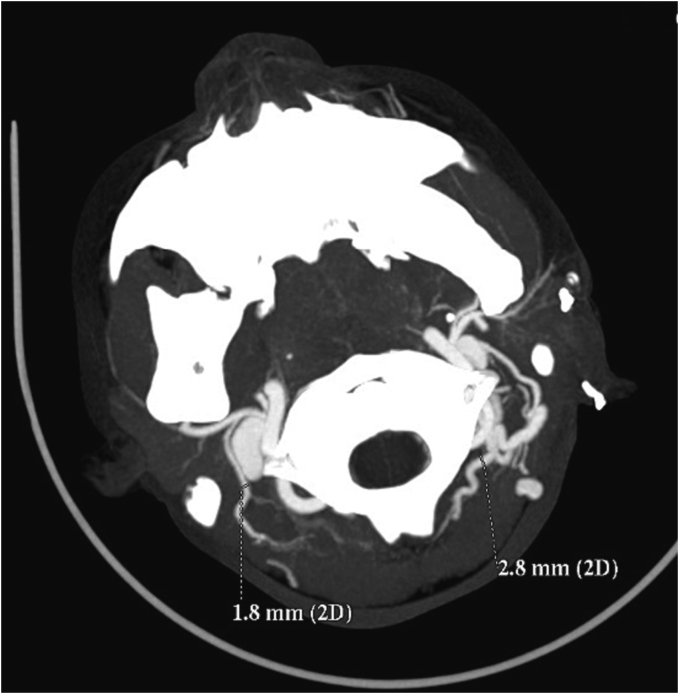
From the CT-Angiography there is different size of internal jugular artery on the right and left side.
